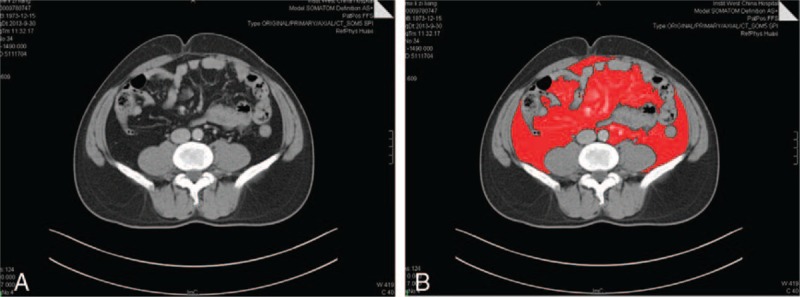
(A) Computed tomography image of umbilicus level and (B) visceral fat area which was measured by Analyze 11.0.
